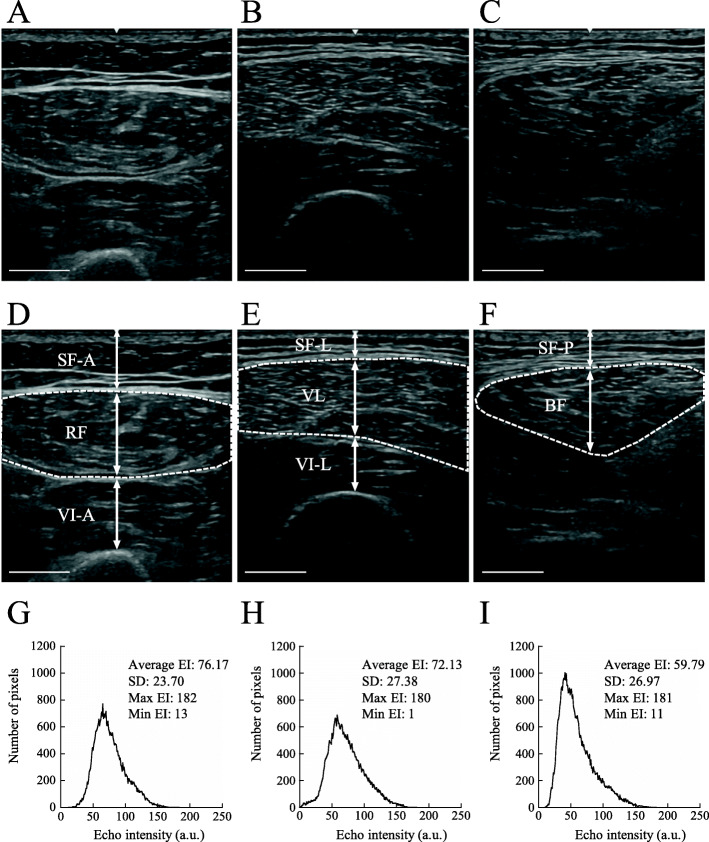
Representative ultrasound images in anterior, lateral, and posterior regions. A and D are the anterior, B and E are the lateral, and C and F are the posterior regions. Surface muscles (rectus femoris from A, vastus lateralis from B and biceps femoris from C) are traced using dotted lines in D, E, and F. Muscle echo intensities, and cross-sectional areas (CSAs) are measured from the dotted-line tracked region. Arrows show the thickness of the subcutaneous fat and muscle. G, H, and I is histograms of the ROI’s pixel intensity from rectus femoris (G), vastus lateralis (H) and biceps femoris (I). SF-A, subcutaneous fat thickness of the anterior region; RF, rectus femoris; VI-A, vastus intermedius of the anterior region; SF-L, subcutaneous fat thickness of the lateral region; VL, vastus lateralis; VI-L, vastus intermedius of the lateral region; SF-P, subcutaneous fat thickness of the posterior region; BF, biceps femoris. White bars are 1 cm in scale
